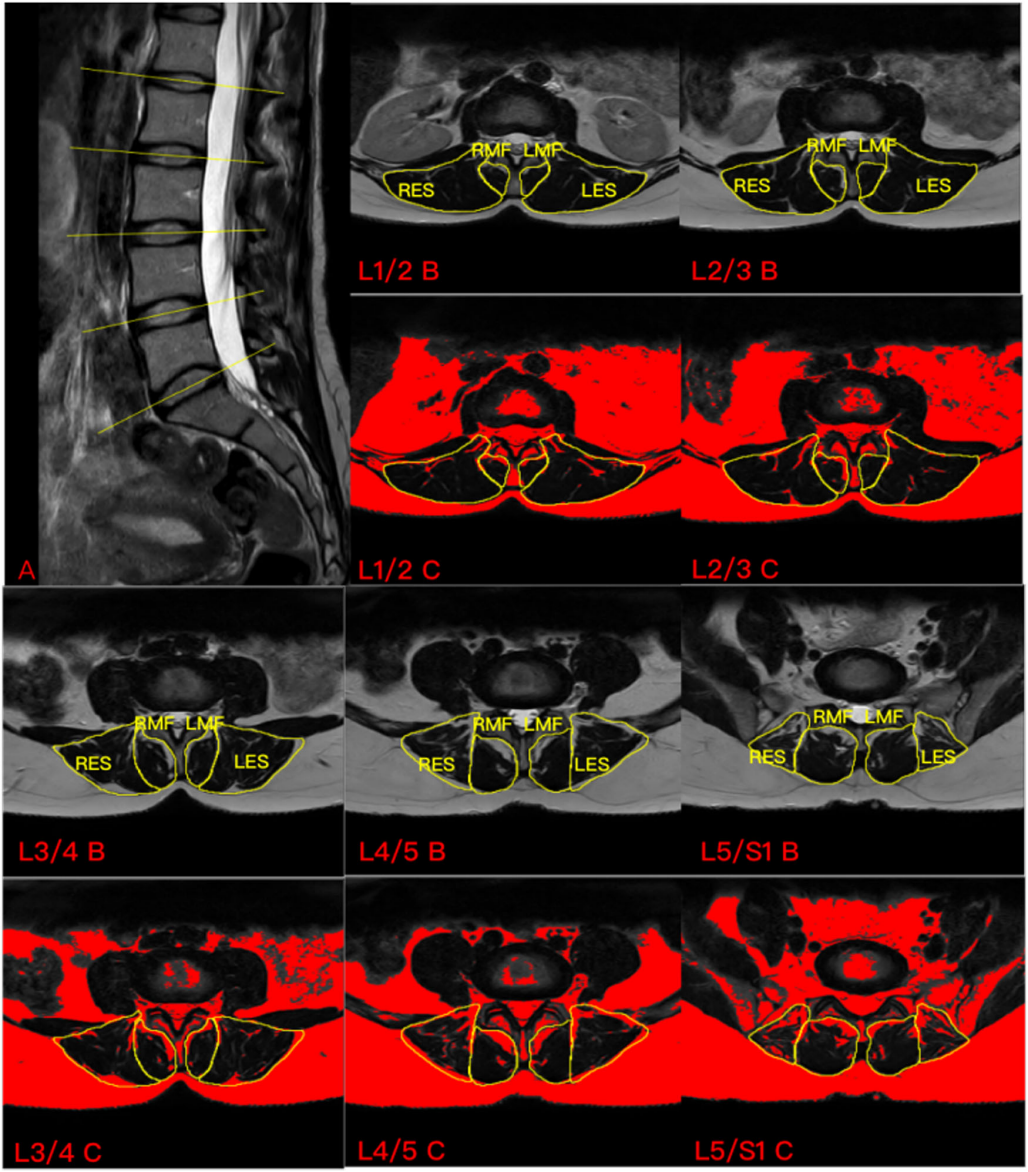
MRI of a subject’s lumbar spine and target muscle measurements. A Sagittal view of the lumbar spine, the yellow line represents the location of the measured cross-sectional MRI. B (L1/2) CSA of the MF and ES at the L1/2 segment. C (L1/L2) FCSA of the MF and ES at the L1/2 segment. LMF, left multifidus; LES, left erector spinae; RMF, right multifidus; RES, right erector spinae
